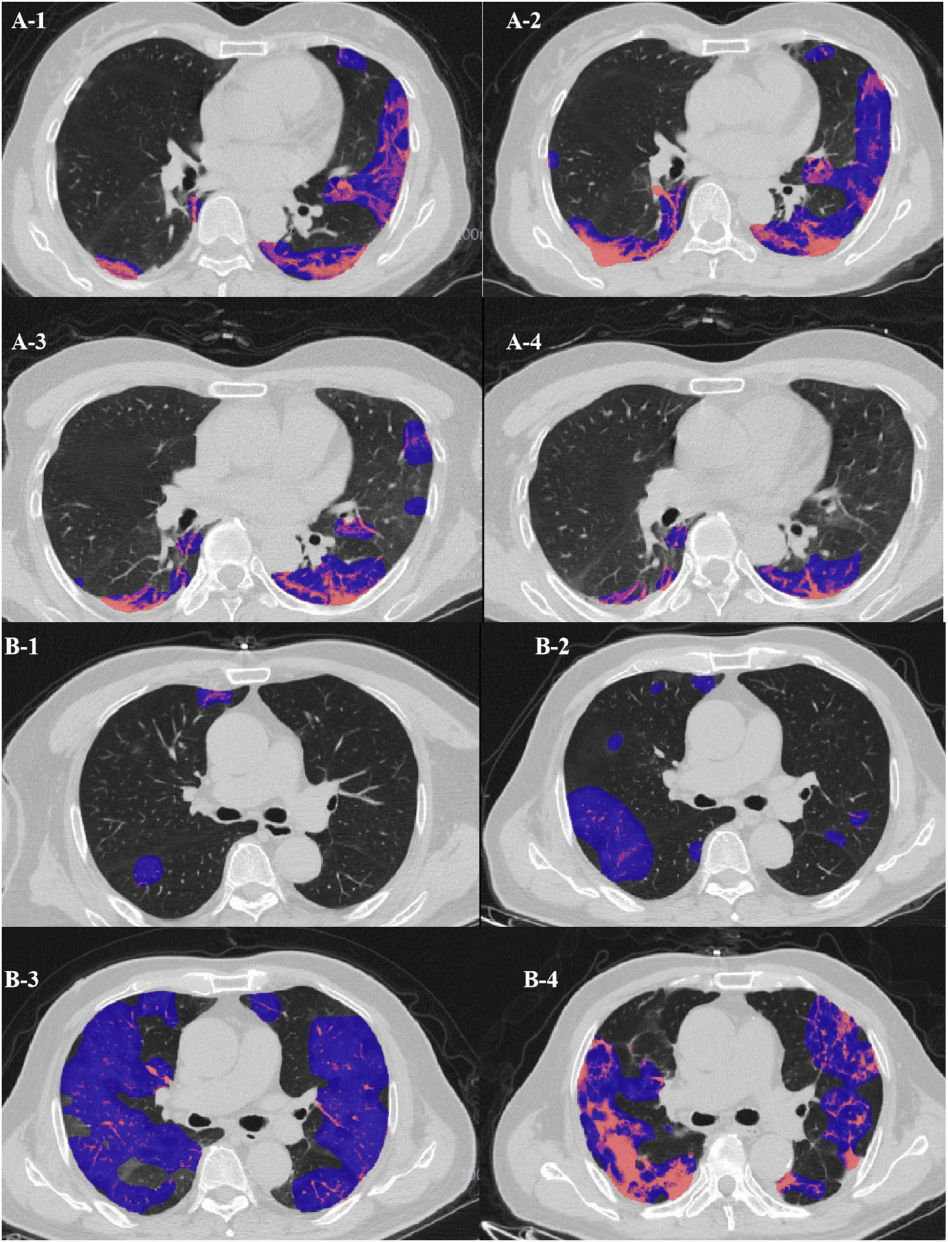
(A) Evolution of CT findings in a 51-year-old female patient of the clinically ordinary type presenting with persistent fever (37.8°C) for 5 days. (A-1) At the presentation (stage 1, day 5), with a PLI of 18.11%, showing a small region of subpleural GGO with partial consolidation mainly in the left upper lobe; (A-2) stage 2 day 10, with a PLI of 25.48%, a region of GGO enlarged while the density decreased; (A-3) stage 3 day 16, the density of the GGO further resolved, with a PLI of 14.71%; (A-4) stage 4 day 28, with a PLI of 8.93%, resolution with minimal residual GGO and parenchymal bands. (B) Evolution of CT findings in a 68-year-old male patient of the clinically severe type presenting with persistent fever (38.3°C) for 3 days, and with coronary heart disease for 5 years. (B-1) At presentation (stage 1, day 2), with a PLI of 2.7%, only small patchy GGO was visible; (B-2) stage 2 day 11, with a PLI of 12.7%, progressed into large patchy region of GGO; (B-3) stage 3 day 18, with a PLI of 57.75%, bilateral “white lung”; (B-4) stage 4 day 23, with a PLI of 48.87%, the “white lung” resolved obviously.
